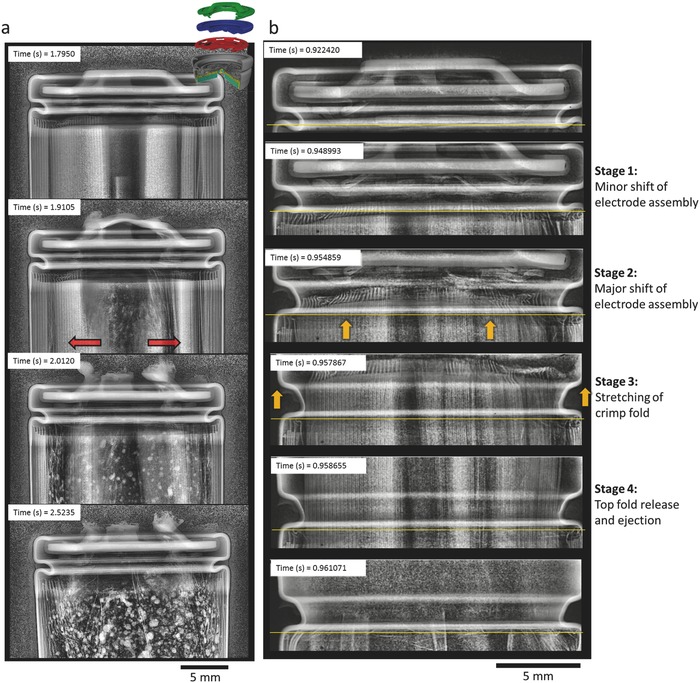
Time‐stamped radiographs taken at a) 2000 fps showing the propagation of thermal runaway within the Samsung cell and the top button melting, and b) taken at 20 272 fps showing the stages of the Samsung cell bursting. The thin yellow line on each image is a reference line at the shoulder of the spin groove. Radiography movies of (a) and (b) are provided as Movies S8 and S10, respectively, in the Supporting Information. The red arrows highlight the radial direction of propagation of thermal runaway, and the yellow arrows highlight the shift of the electrode assembly and extension of the spin groove.
